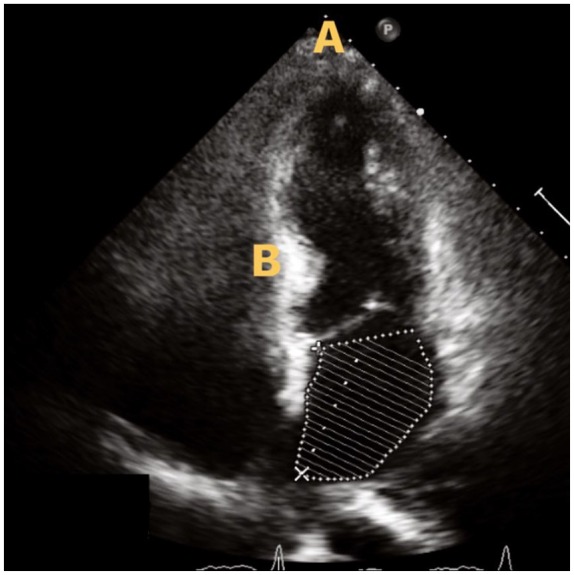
Echocardiogram reveals apical akinesis (A) and basal hyperkinesis (B)
